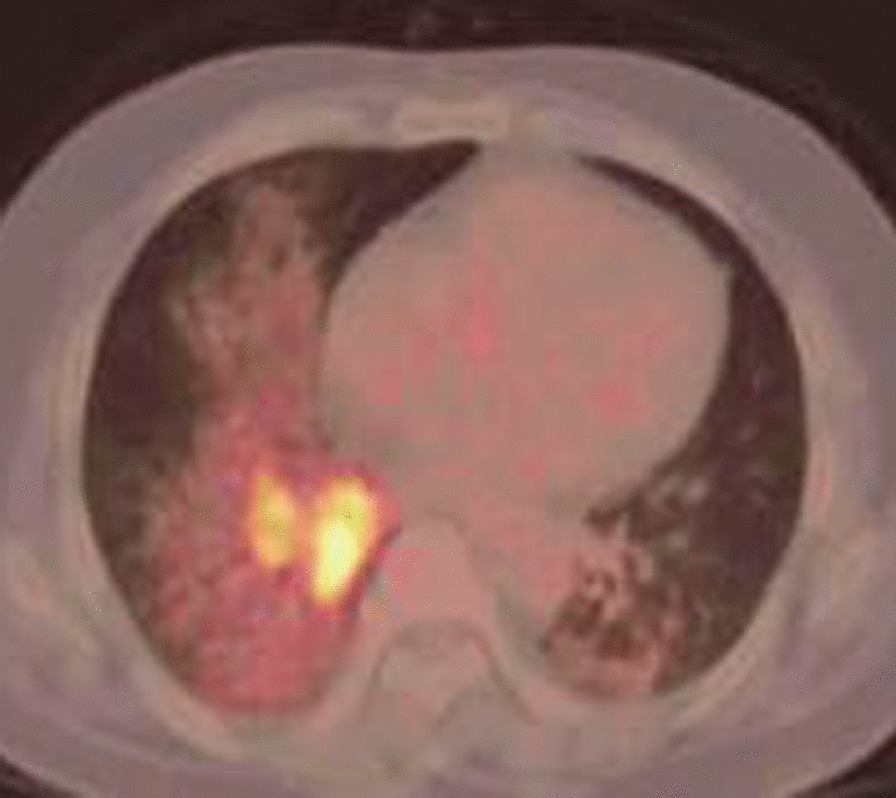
PET-CT scan showing patchy consolidation in the basal segment of the right lower lobe. PET-CT scan shows FDG metabolism was abnormally increased. FDG, fludeoxyglucose
